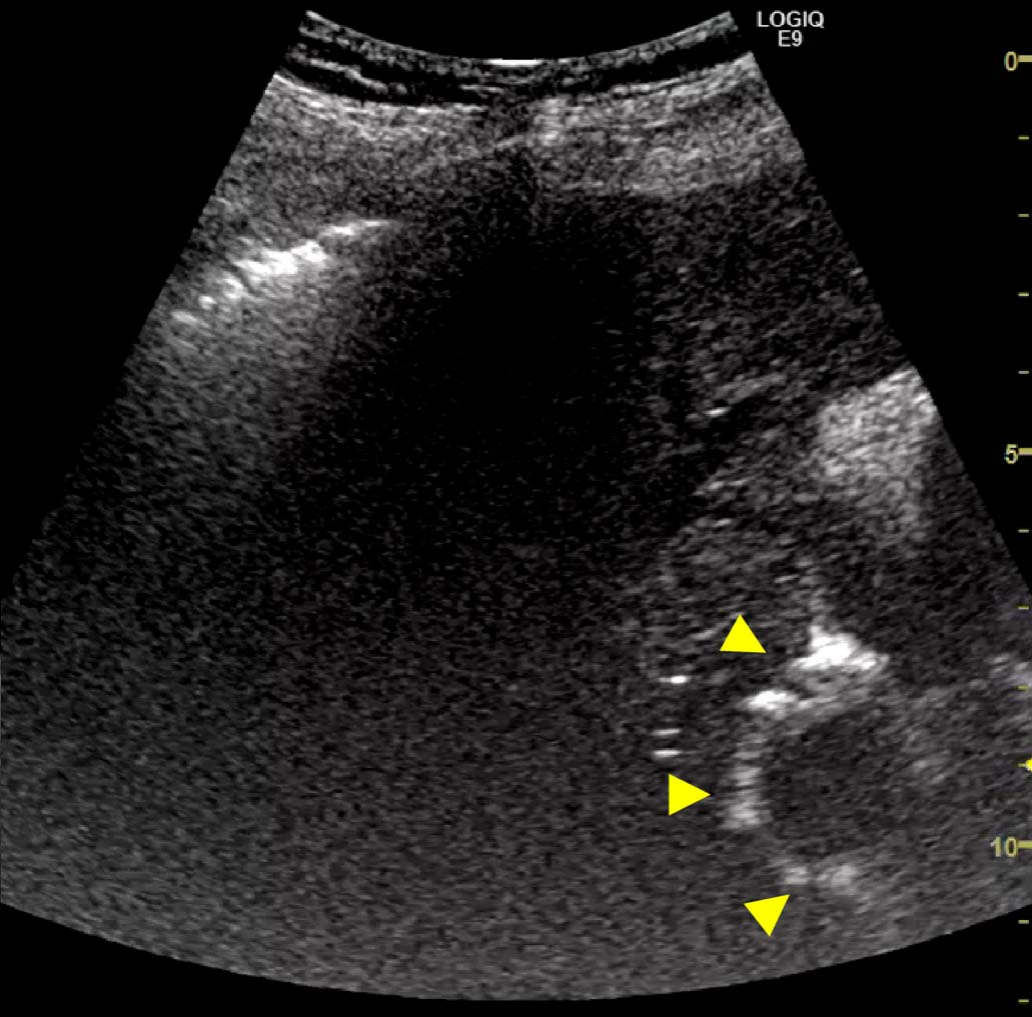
Ultrasound confirming enhancement of the peripheral bile duct close to the lesion with no bile duct injury (yellow arrowheads).
